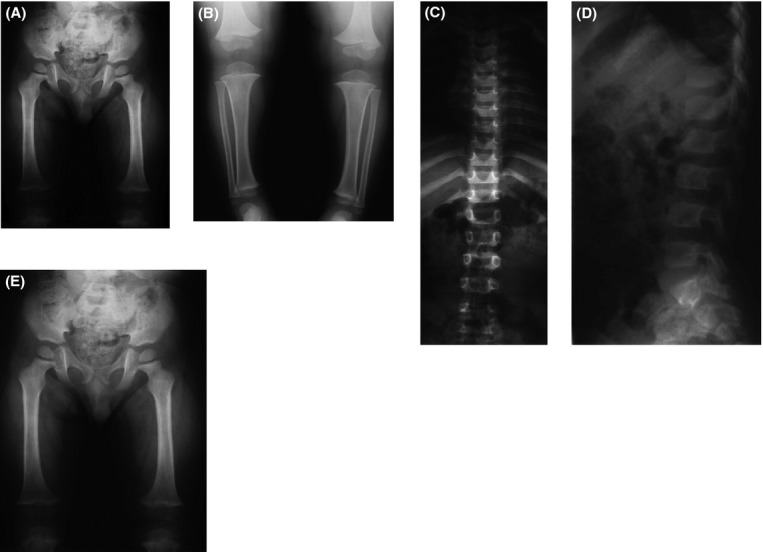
Radiographs of a case of achondroplasia with a p.Asn540Lys mutation (R#00-347). (A) Pelvis and femur, age 3 years. Narrow sacrosciatic notches and femoral shortening. (B) AP lower legs, age 3 years. There is distal overgrowth of the fibulae. (C) AP spine, age 3 years, shows platyspondyly and interpediculate narrowing in the lumbar spine. (D) Lateral spine, age 3 years. Note a narrow spinal canal and a wedge vertebrae at L2. (E) PA, left hand, age 3 years. There is significant brachydactyly and brachymetacarpia.
